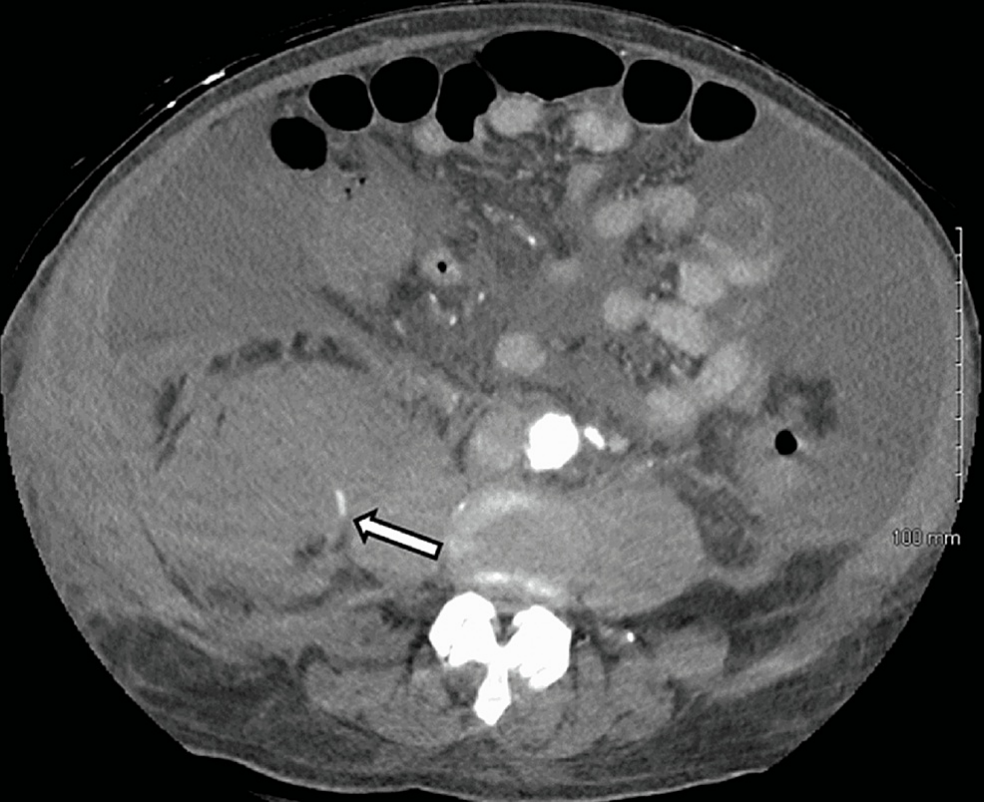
CT angiogram showing contrast extravasation (arrow) within a large right retroperitoneal hematoma, indicating active bleeding
